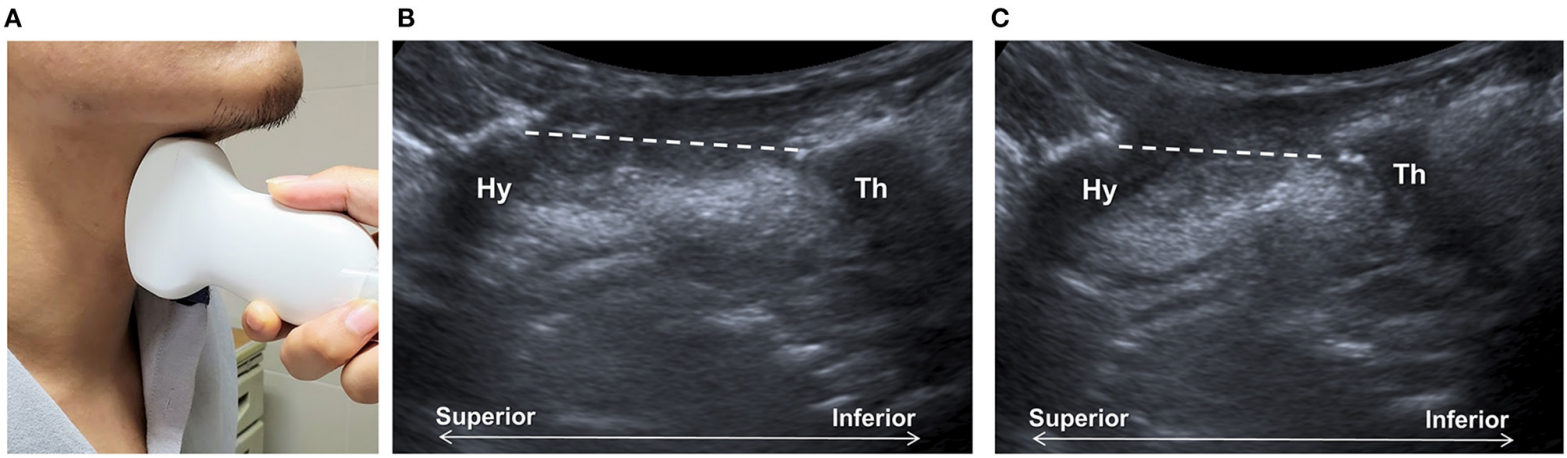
Ultrasonography assessment of thyroid-hyoid approximation. The transducer is placed at the midsagittal plane at the anterior neck (A), between the thyroid cartilage (Th) and the hyoid bone (Hy). Sonographic imaging demonstrates the measurement of distance (dashed line) between the thyroid bone and thyroid cartilage at the resting position (B) and at maximal approximation (C).
